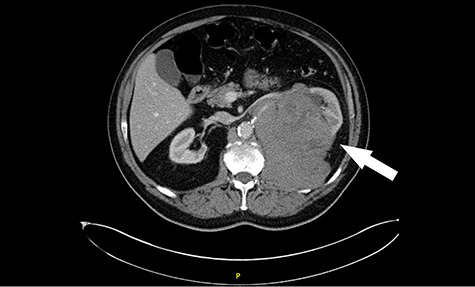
Computed tomography (CT) revealing a large insinuating left renal soft tissue mass measuring 140 × 105 mm.
